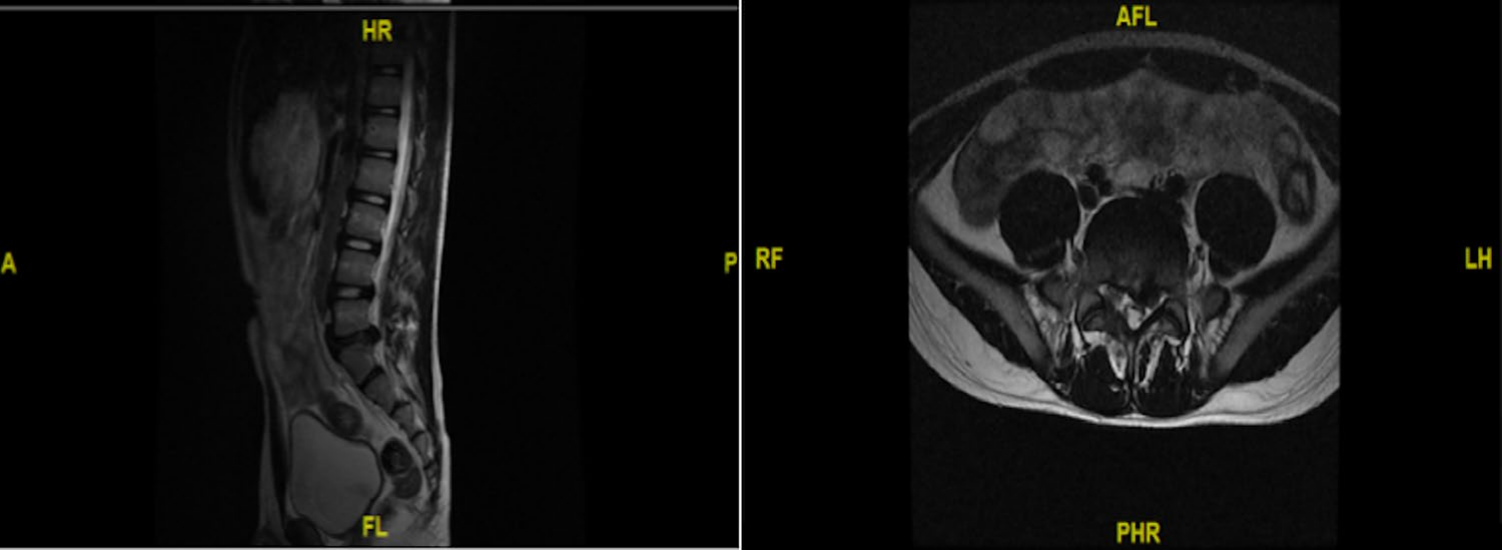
Asymptomatic residual disc tissue was observed on MRI 3 months after operation
